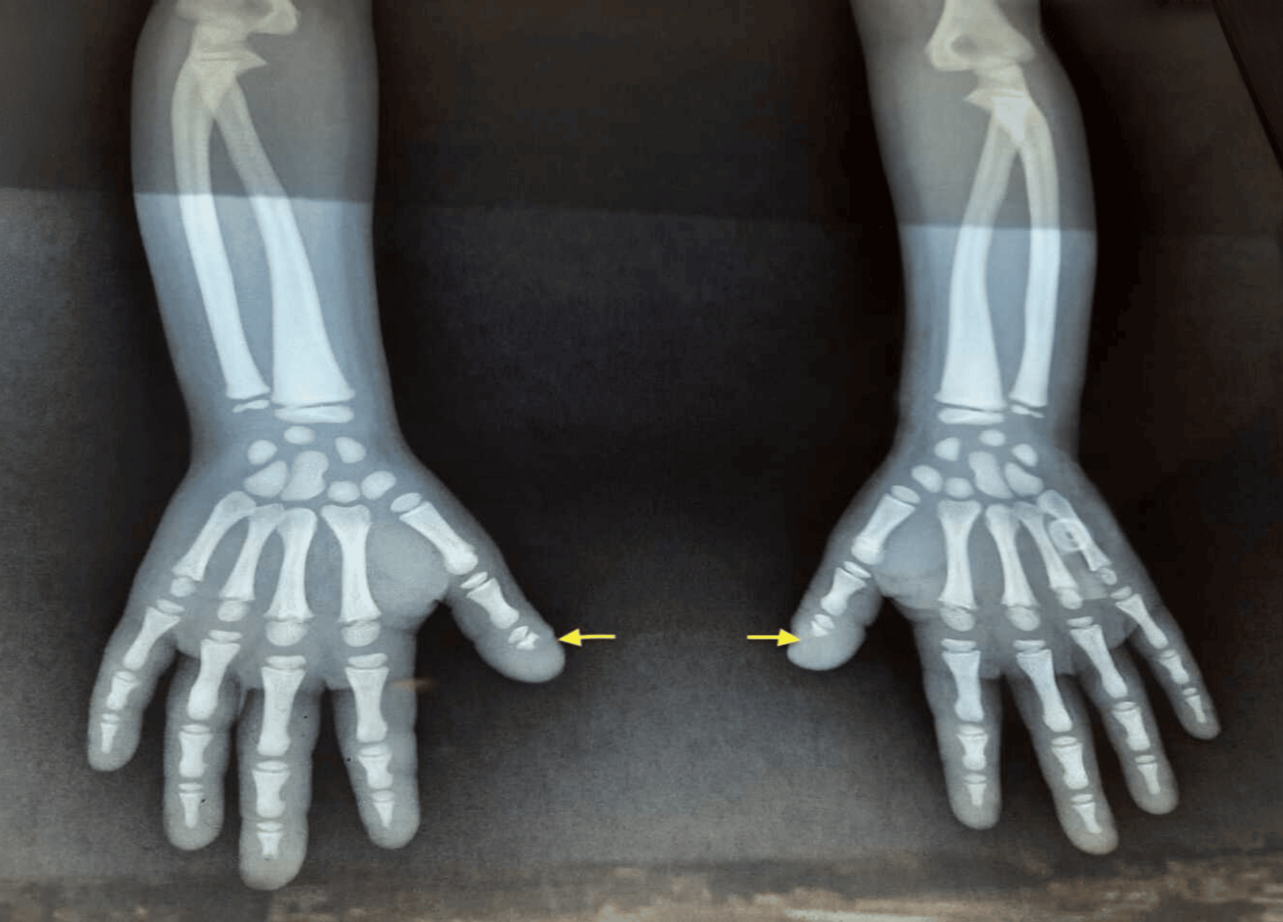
Radiograph of the both hands showing acro-osteolysis (arrow)
